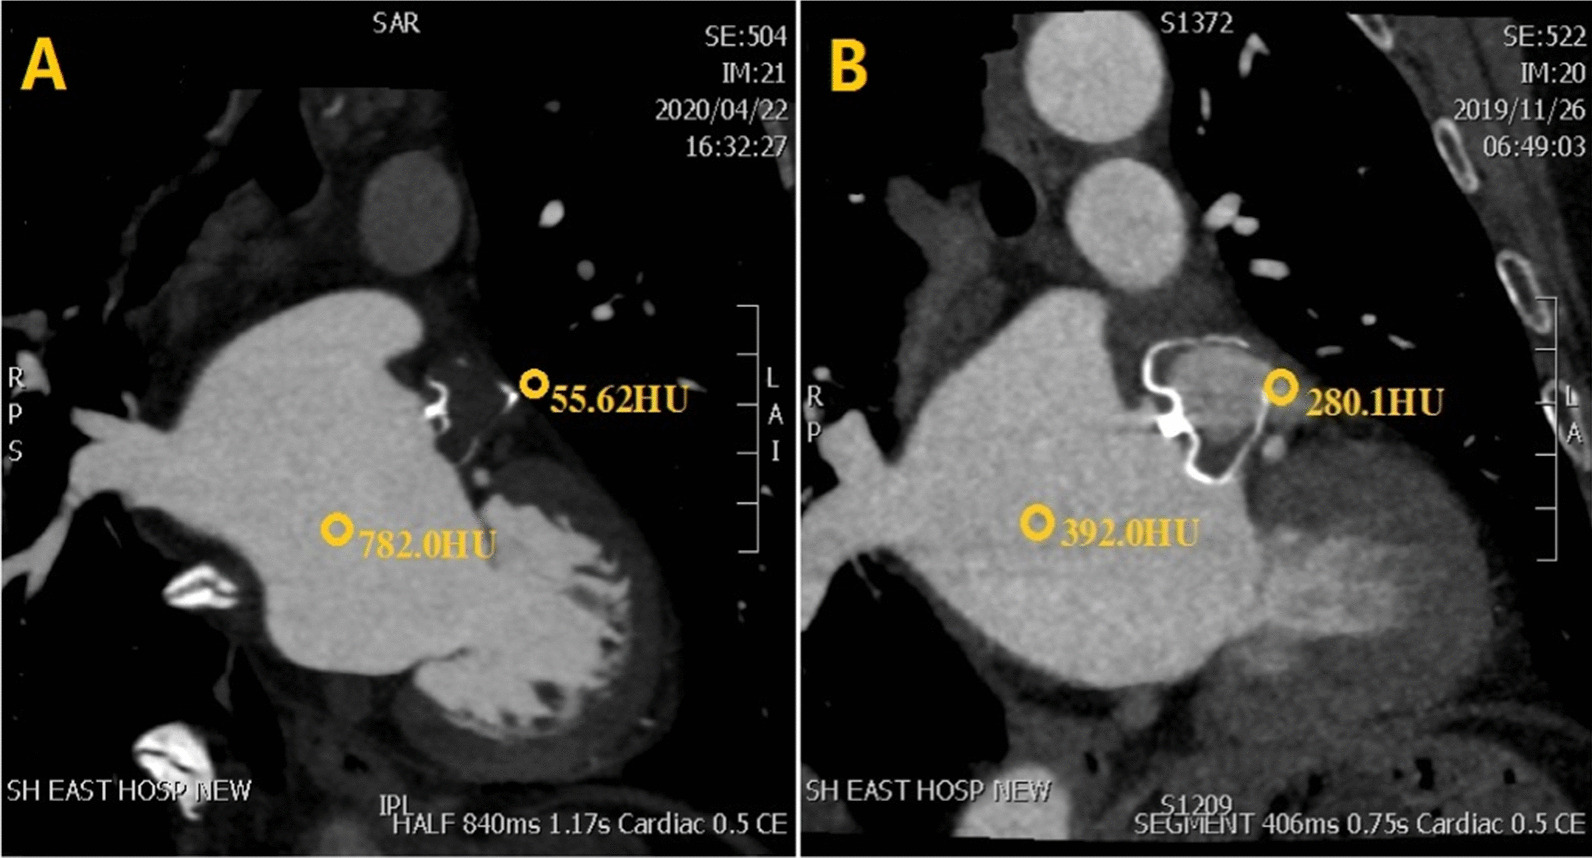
Watchman device endothelialization assessed by cardiac CT. a complete occlusion of the left atrial appendage (no trans-fabric leak, LAA HU < 100 and LA/LAA < 0.25). b Incomplete endothelial coverage of the device (trans-fabric leak, LAA HU > 100)
